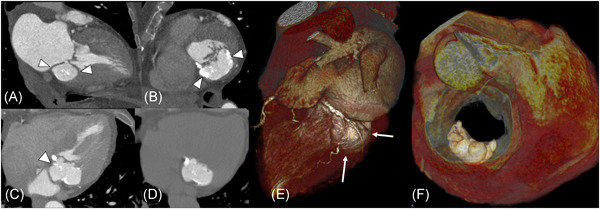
Contrast‐enhanced CT images (A–C) showed a 50 × 43 mm hyperdense crescent‐shaped mass located within a bi‐lamination of the posterior mitral annulus(arrowheads) in a 82‐year‐old woman. Noncontrast CT images (D) excluded diagnosis of pseudoaneurysm, by revealing homogeneous caseous content with some calcifications. On 3D‐volume‐rendered images the lesion mimics ventricular outpunching (E) and appears nonobstructing trans‐mitral flow (F). CT, computed tomography
